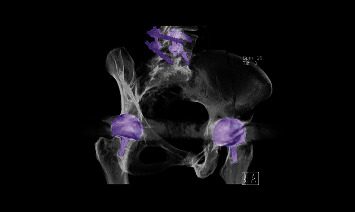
CT volume rendering of the pelvis. The reconstruction of all CT planes gives an overview of the fracture of the Os ilium and the extent of the cyst.
